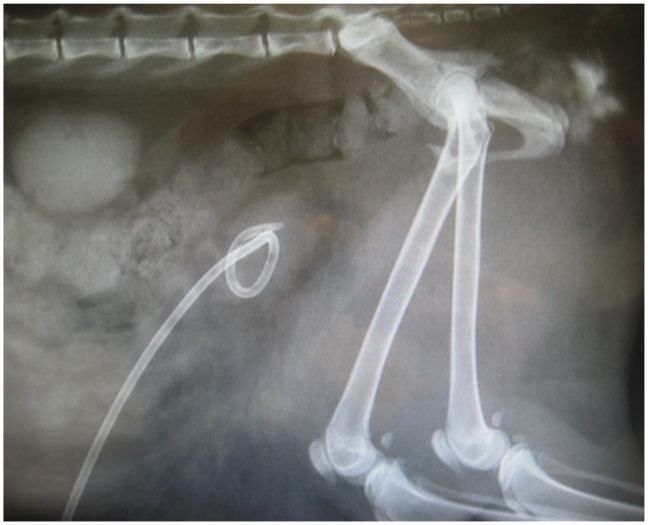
Lateral radiograph of the abdomen showing the pigtail catheter located in the bladder
